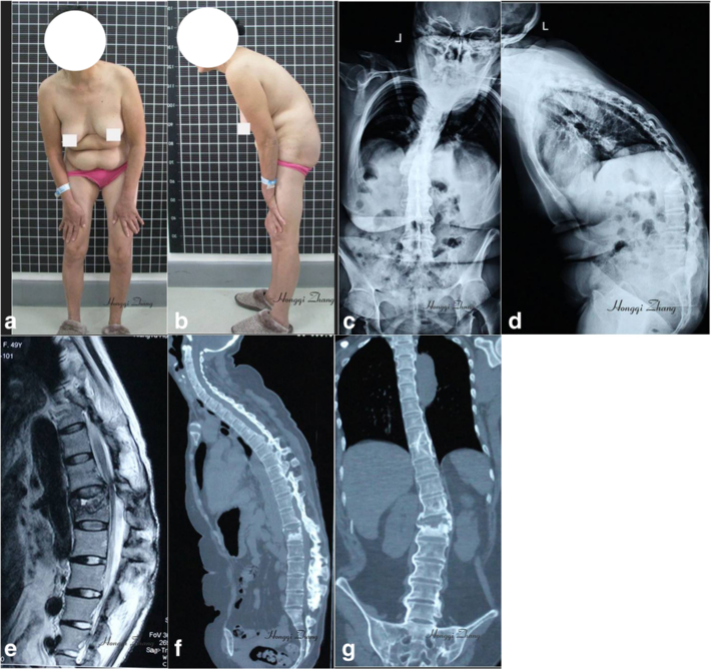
Preoperative imaging findings of a 47-year-old female patient with ankylosing spondylitis. a, b Photographs show a kyphotic deformity, with the patient having difficulty in holding the head up straight. c, d Radiographs show the thoracolumbar kyphotic deformity, with a pathological fracture located at L1. e MRI image shows destruction of bone and compression of spinal cord. f, g CT images show the pathological fracture at L1
